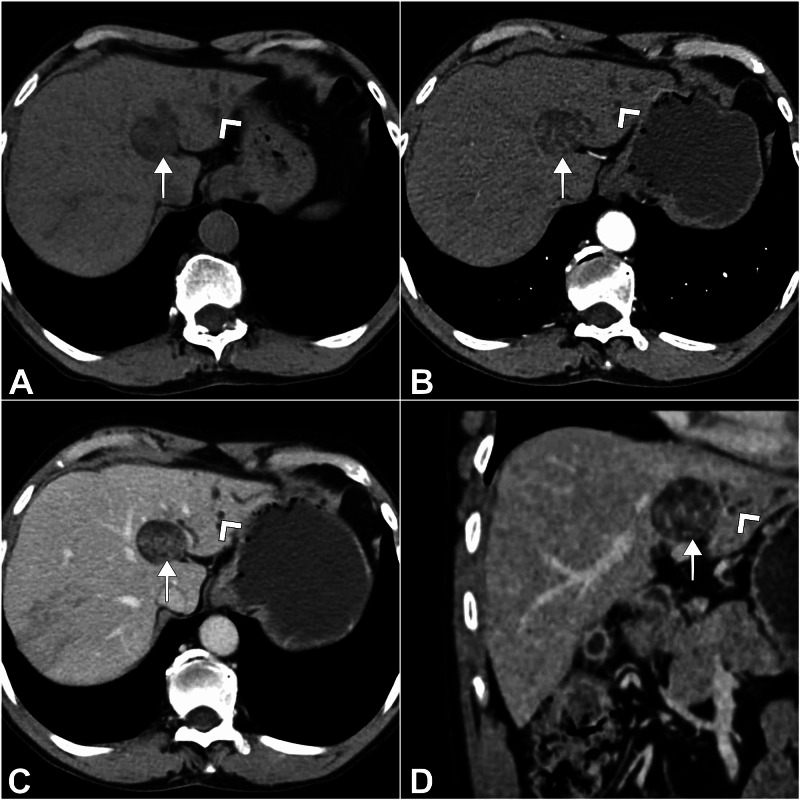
CT images. IPNB lesion is marked with a white arrow. Dilated left-sided biliary radicles are shown with white arrowheads. (A) Axial section, non-contrast (plain) phase; (B) axial section, arterial phase; (C) axial section, portal phase; (D) reconstructed coronal section, delayed phase. CT, computed tomography; IPNB, intraductal papillary neoplasm of the bile duct
